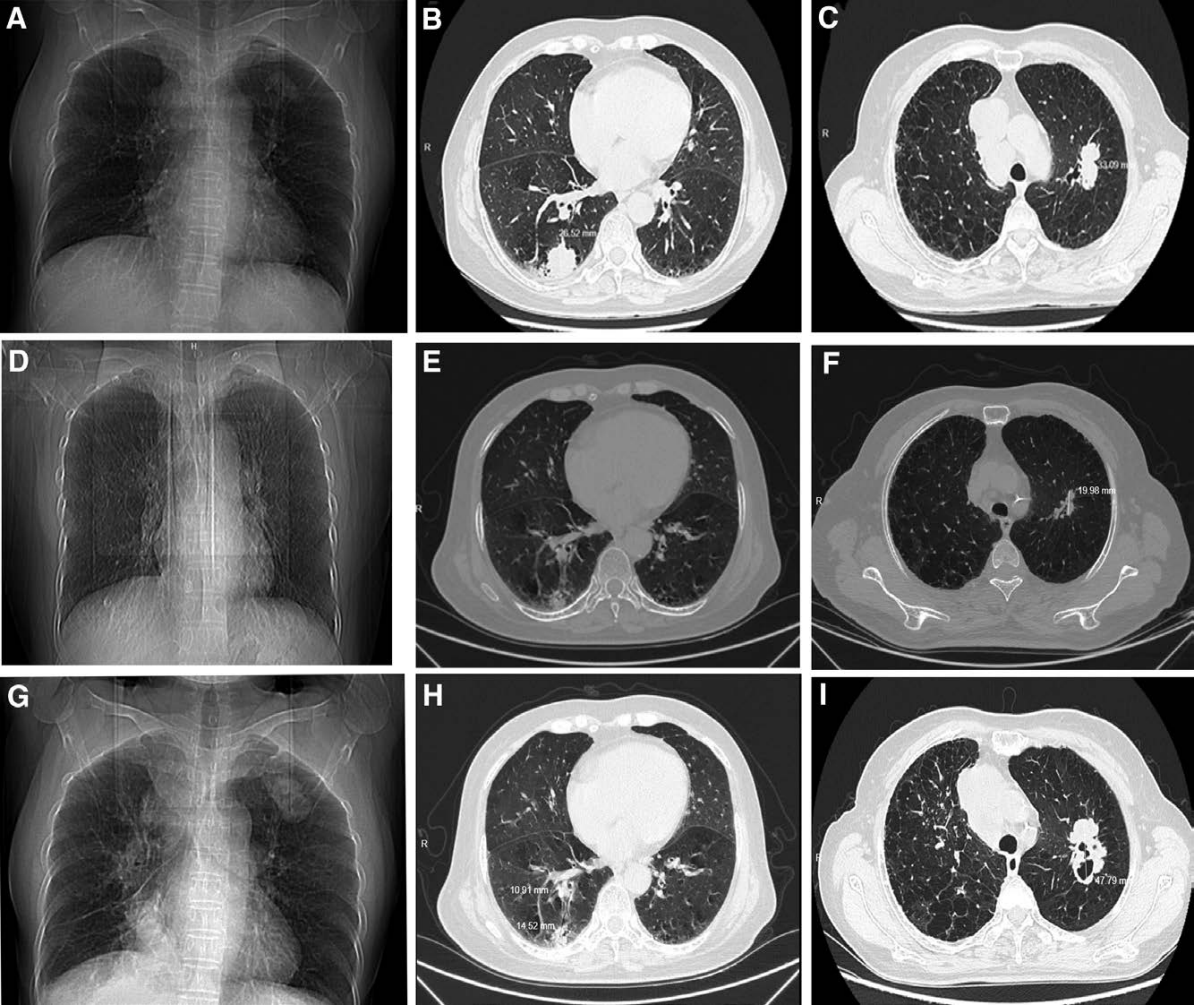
Chest computed tomography (CT) scans of a 65-year-old female patient before and after treatment with ICIs. (A–C) Pretreatment chest CT (August 30, 2021); (D–F) Posttreatment chest CT (December 06, 2021); (G–I) Chest CT after 9 months of treatment interruption (September 14, 2022).
